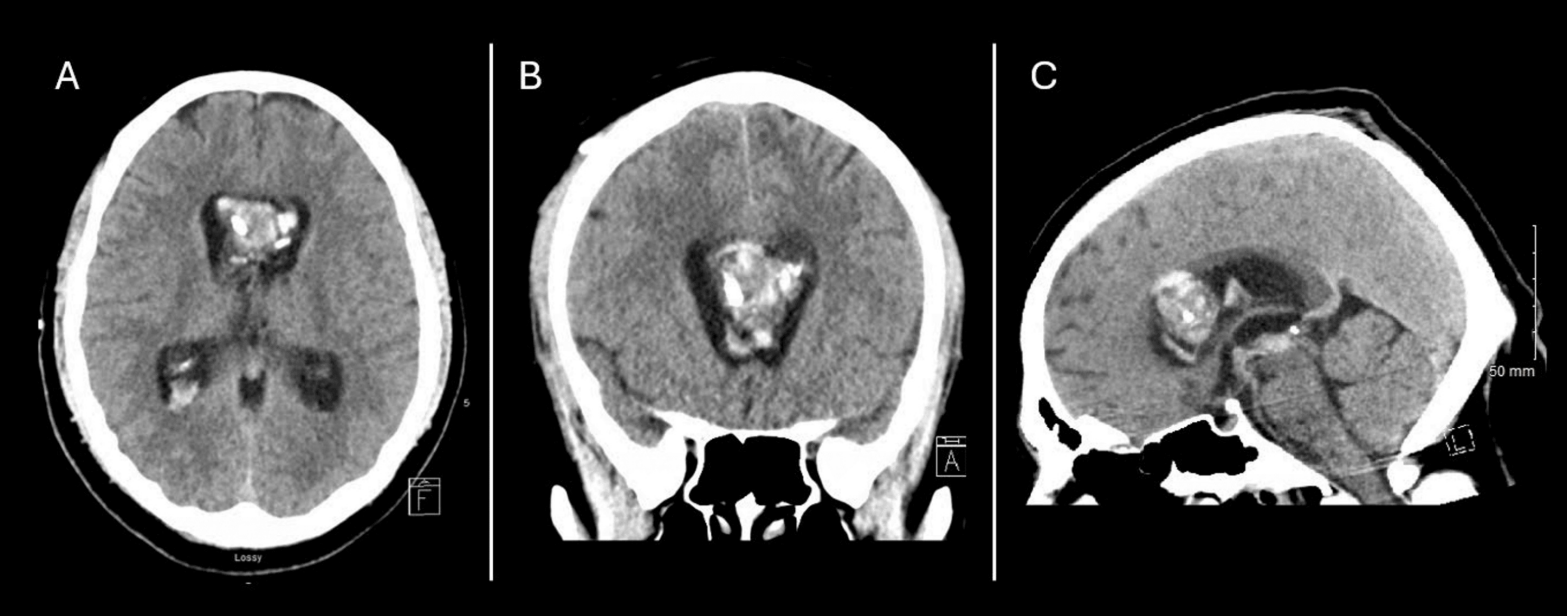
Initial CT Imaging Demonstrating Intraventricular Hemorrhage with Obstructive Hydrocephalus Initial brain CT scan shows intraventricular hemorrhage in the lateral ventricles with associated ventriculomegaly. An external ventricular catheter is also observed in the right frontal horn, in direct contact with the tumor mass (A and B). Obstruction at the level of the cerebral aqueduct due to intraventricular hemorrhage is seen (C).
